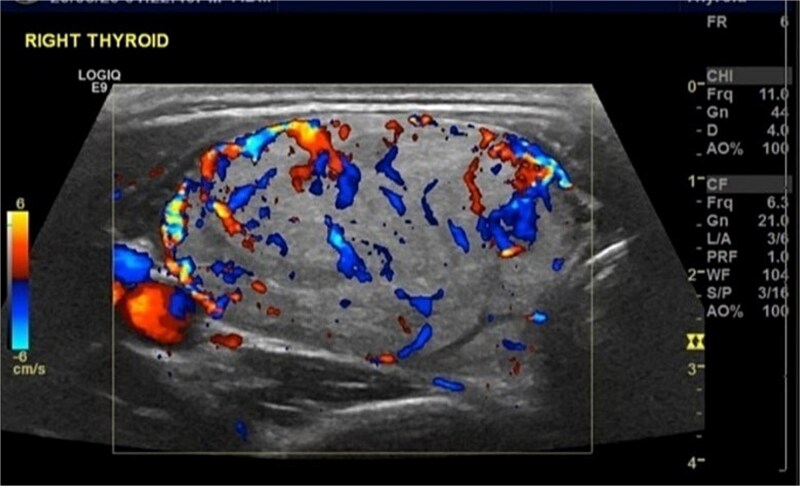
Thyroid color Doppler shows multiple oval isoechoic solid nodules are observed within the enlarged right thyroid lobe, with the largest measuring ~40 × 25 mm with perinodular vascularity.
